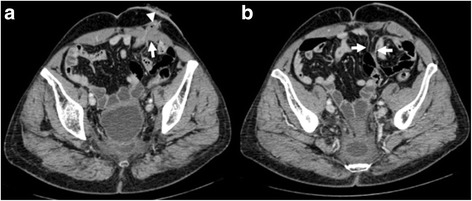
Axial abdominal CT images with a post-contrast portal phase. a Normal appearance of colostomy (*arrowhead*) with the adjacent mesh partially observed. In a previous slice b it is appreciated the funnel of the mesh oriented to the abdominal cavity
